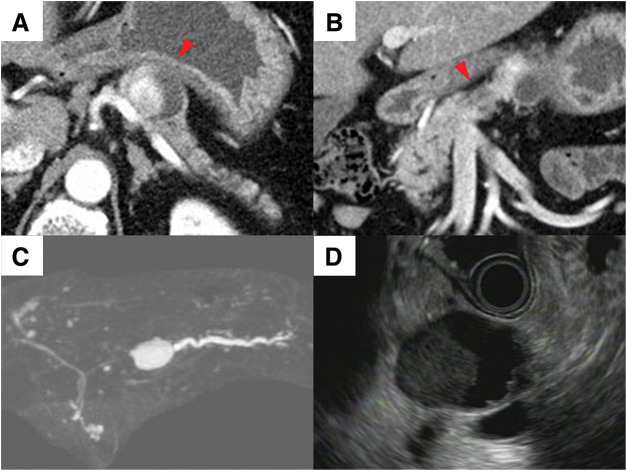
Imaging findings. (A) Axial contrast-enhanced CT showing a cystic lesion protruding outside the pancreas in the tail region, containing a 26-mm enhancing solid component (arrowhead). (B) Coronal CT showing atrophy of the pancreatic parenchyma proximal to the lesion (arrowhead). (C) Magnetic resonance cholangiopancreatography demonstrating a cystic lesion in the pancreatic tail with dilatation of the distal pancreatic duct. (D) Endoscopic ultrasonography showing a unilocular cyst with a localized solid component in the pancreatic tail.
